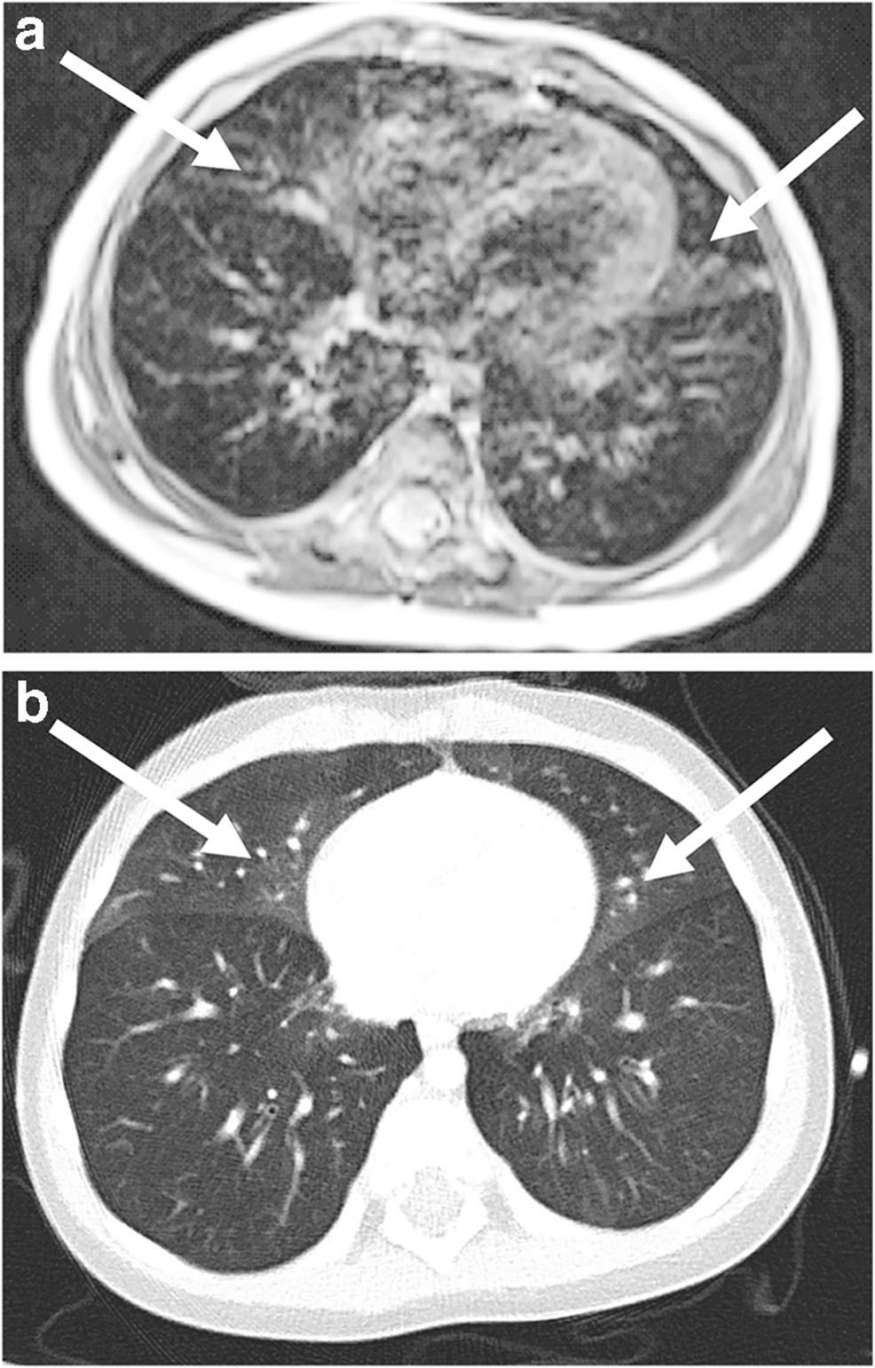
Diagnosis of neuroendocrine cell hyperplasia in an 8-month-old girl. a Axial T2-weighted turbo spin-echo MR image (parameters in Table 2) shows multisegmental interstitial ground-glass opacities in the right middle lobe and in Segment 5 of the left lung (*arrows*). The findings are not very pronounced. MR diagnosis of the condition should not yet be recommended in our experience. b Corresponding CT image shows subtle ground-glass opacities (*arrows*)
